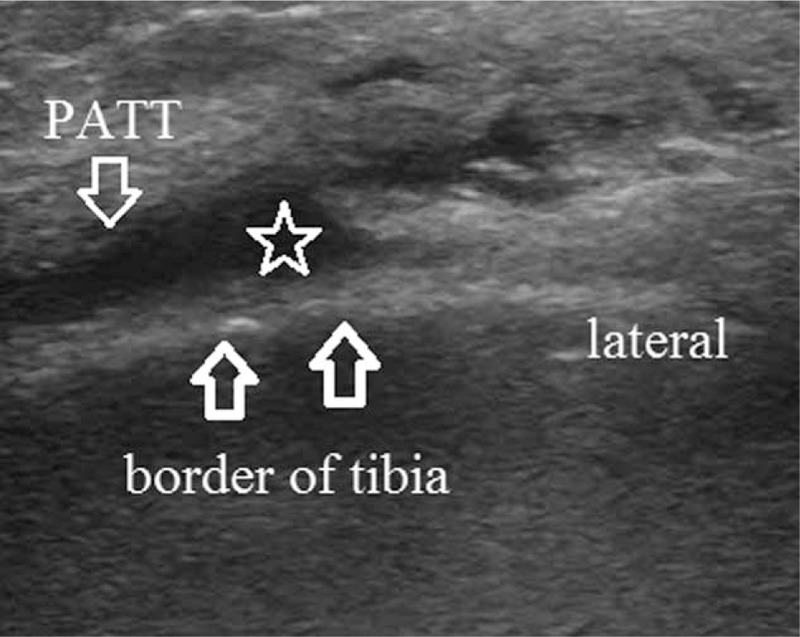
Ultrasound image showing increased fluid collection within the pes anserine bursa (asterix). PATT = pes anserine tendon thickening.
